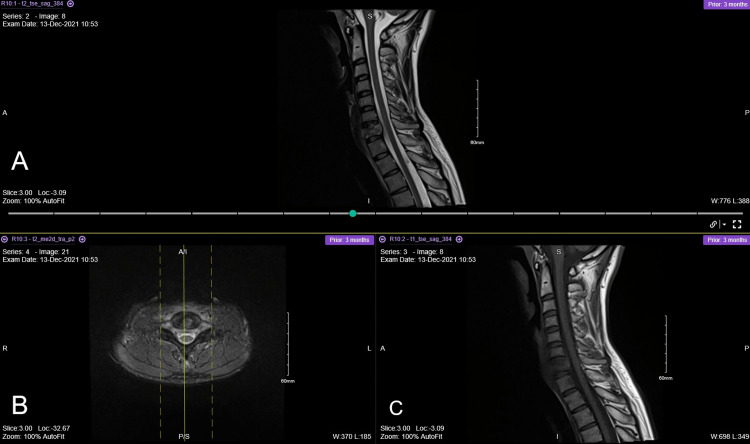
Cervical spine MRI done outside shows C7 destructive lesion with collection size extending from the level of C5 to upper border of T3 anteriorly. (A) T2 sagittal view, (B) axial view, and (C) T1 sagittal view.
